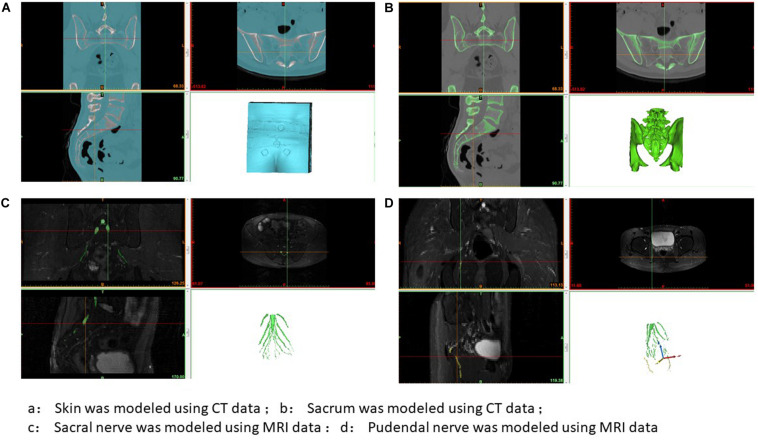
3D printing guide plate data extraction. (A) Skin was modeled using CT data; (B) Sacrum was modeled using CT data; (C) Sacral nerve was modeled using MRI data; (D) Pudendal nerve was modeled using MRI data.
